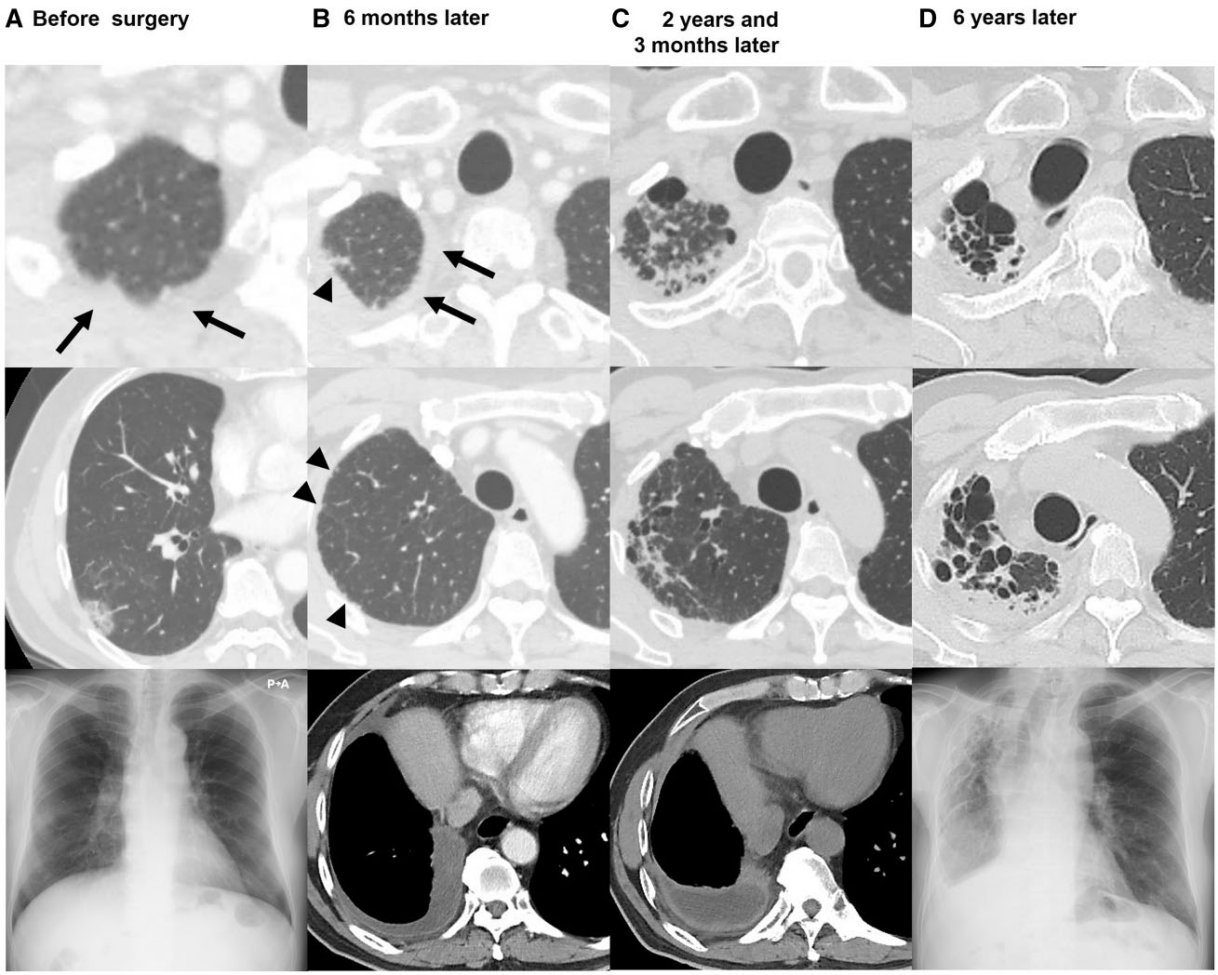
Radiological course of a 68-year-old male patient who underwent right lower lobectomy for stage IA adenocarcinoma. (A) Before surgery, computed tomography showed a pulmonary apical cap (arrow) and a mixed ground-grass opacity in the right lower lobe. A chest radiograph showed a ground-grass opacity in the right middle field of the lung. (B) Postoperative computed tomography 6 months after surgery demonstrated involvement of the pleura (arrow) and subpleural parenchyma (arrowhead) in the right upper lobe and right pleural effusion. (C) At 2 years and 3 months after surgery, a subpleural parenchymal lesion in the right upper lobe appeared to be deteriorated with cystic change and thickened pleural effusion. (D) At 6 years after surgery, the cystic lesion in the right upper lung field was obviously deteriorated with unilateral thoracic deformity.
